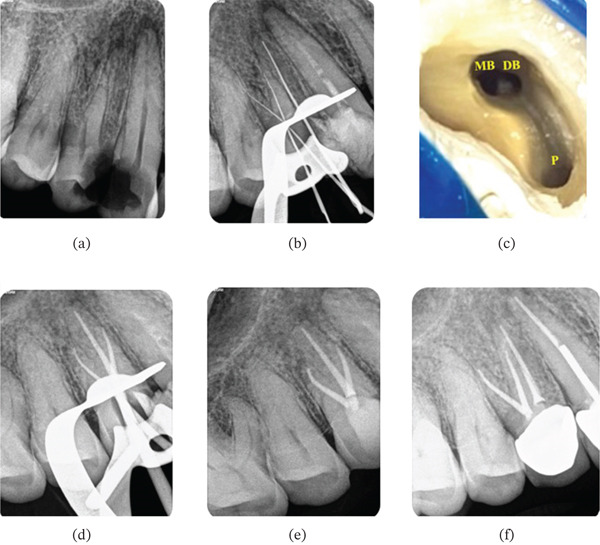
(a) Preoperative radiograph in relation to 14 and 13; (b) working length radiograph in relation to 14; MB = 19 mm, DB = 19 mm, and P = 21 mm; (c) T‐shaped access cavity showing three separate orifices under magnification; (d) master cone radiograph in relation to 14; (e) obturation and core build up radiograph in relation to 14; and (f) 1 year follow‐up radiograph with evidence of periapical healing in 14 and 13 with post endodontic PFM full coverage crowns.
